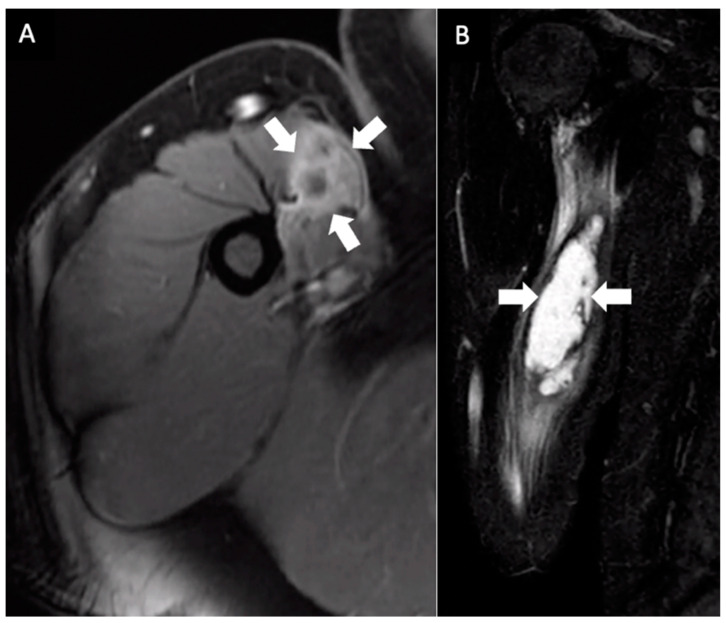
Multiplanar MRI of the right upper arm taken one month after identifying the mass and seven months after receiving the booster dose. Axial fast spin gradient echo postcontrast (A) and coronal STIR (B) images show an ill-defined central fluid signal measuring 10 × 3.1 × 1.9 cm with prominent peripheral enhancement (arrows).
